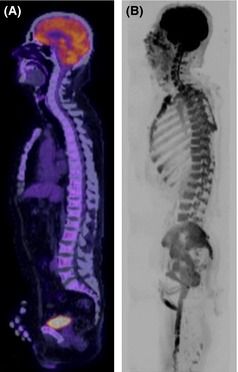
Diffusion weighted magnetic resonance imaging (DW‐MRI) shows increased sensitivity for diffuse marrow infiltration. Sagittal ^18^F‐fluorodeoxyglucose positron emission tomography (^18^F‐FDG PET)/computerized tomography (CT) (A) and b900 whole body DW‐MRI Maximum Intensity Projection image in a 52‐year‐old male with multiple myeloma. (A) ^18^F‐FDG PET/CT was reported as normal with no areas of increased FDG uptake and no lytic lesions on the CT component. (B) Inverted greyscale WB DW‐MRI demonstrated diffuse marrow infiltration. Marrow trephine confirmed 80–90% infiltration with plasma cells.
